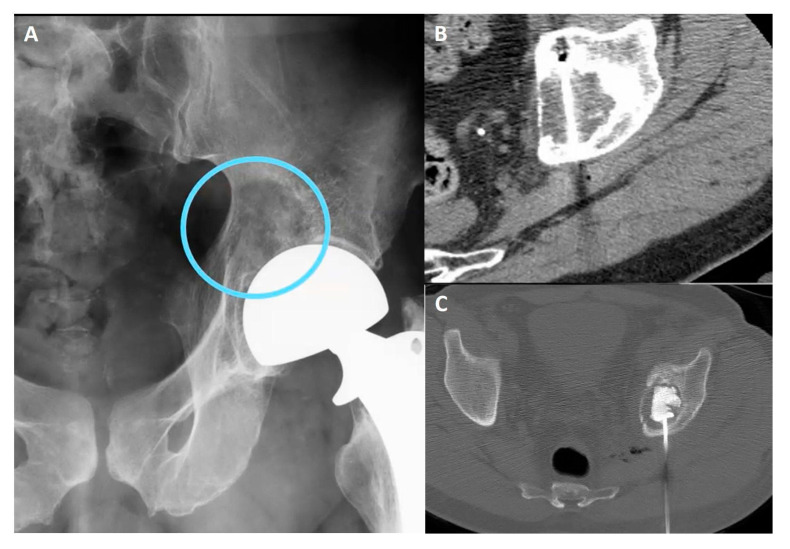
(A) Pelvic X-ray showing osteolytic lesion of left periacetabular region (circle). (B) Axial CT scan showing cryoablation needle inserted in the osteolytic lesion. (C) Axial CT scan showing postcryoablation cementoplasty performed to augment the ablative results and to prevent bone fractures.
